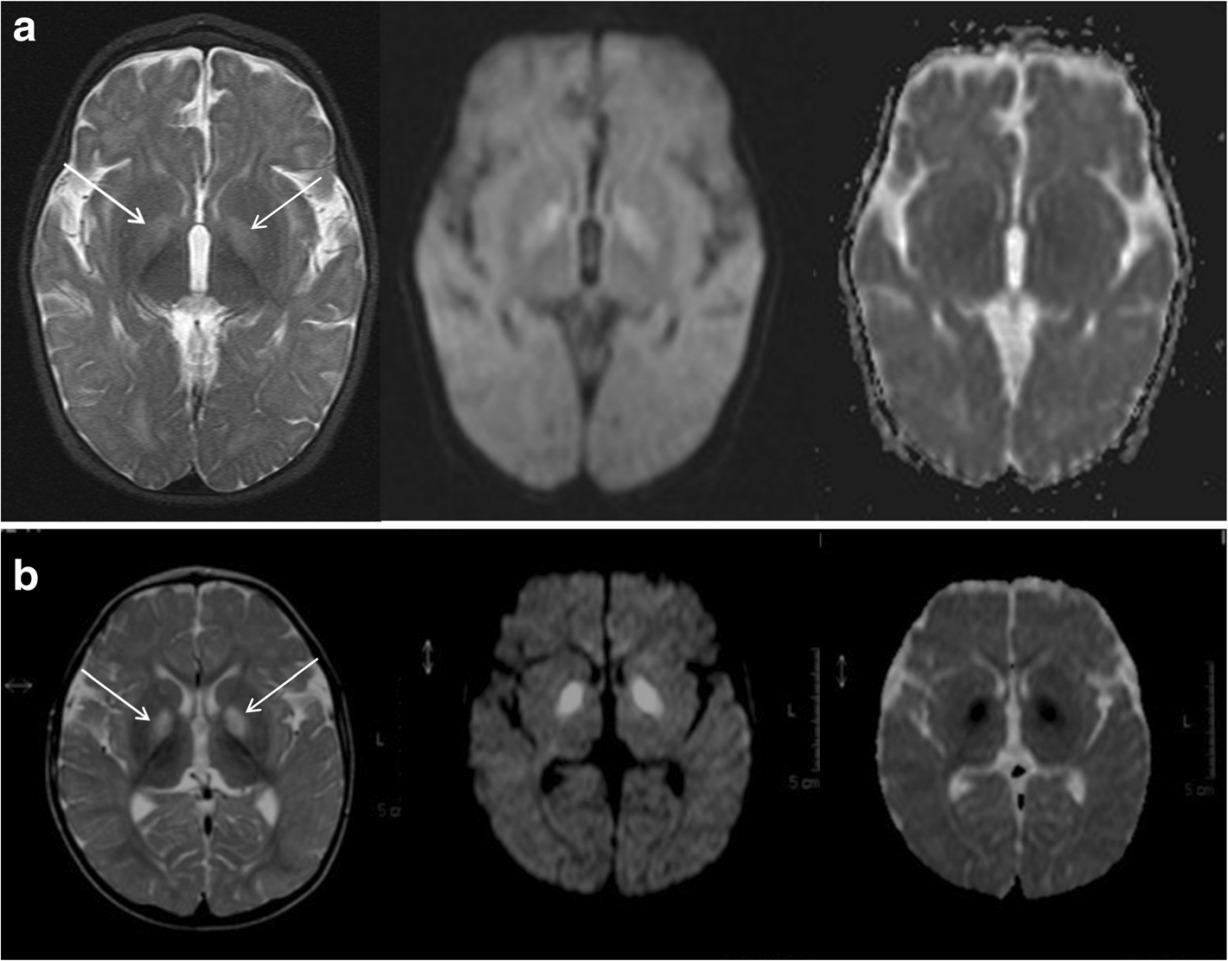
Magnetic resonance imaging of the brain in HIBCH deficiency. (a) Patient 2 at 9 months: axial T2 weighted image (left panel) shows bilateral symmetrical signal hyperintensity within the globi pallidi (arrows) accompanied by signal hyperintensity on diffusion weighted image (middle panel) and low signal on ADC map (right panel) in keeping with restricted diffusion which is beginning to pseudonormalise on ADC map. The features are those of a subacute neurometabolic insult. In addition there is some generalised non-specific lack of cerebral volume with prominence of the cerebral sulci and delay in myelin maturation. (b) Patient 3 at 11 months: axial T2 (far left) shows bilateral symmetrical signal hyperintensity and swelling in the globi pallidi (arrows) with restricted diffusion (diffusion weighted image middle panel, ADC map right panel) consistent with cytotoxic oedema. The imaging pattern is suggestive of an acute neurometabolic insult.
